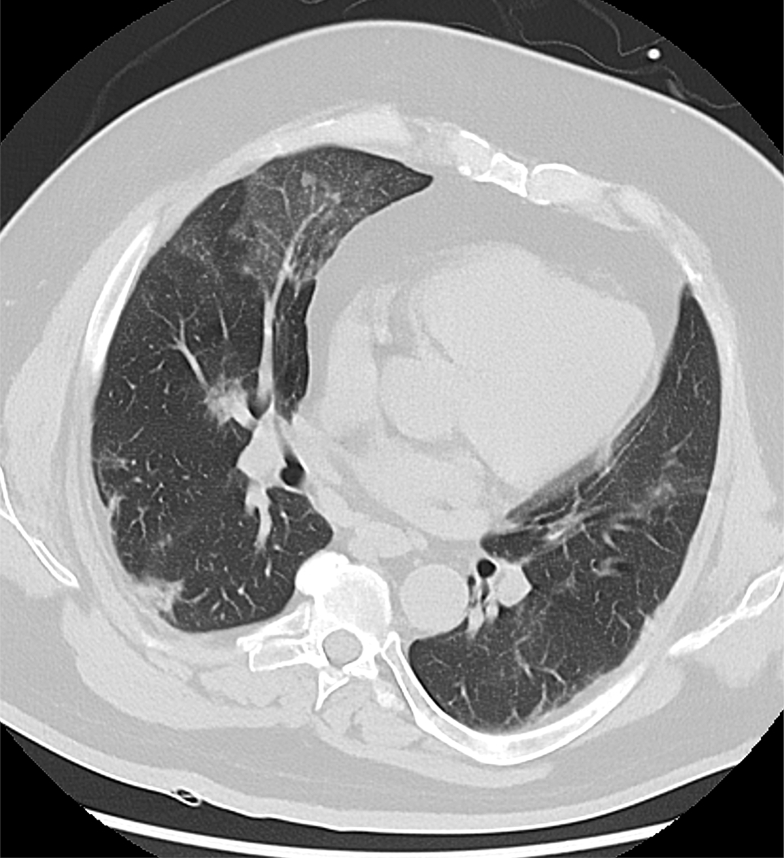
Parenchymal changes in the right middle and both lower lobes suggest COVID-19-pneumonia (previous CT scan from 2020).
